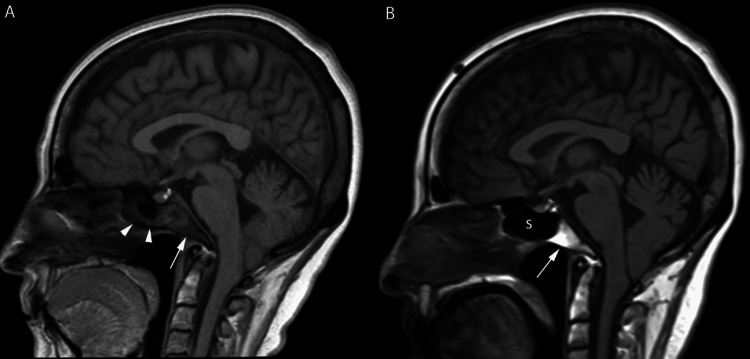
Magnetic resonance imaging of the head Sagittal T1 weighted images (A) demonstrate mild mucoperiosteal thickening in the sphenoid sinus (arrowheads in A) with abnormal signal intensity within the adjacent clivus (arrow in A). The abnormal signal intensity in the clivus becomes more apparent (arrow in B) with no significant sinus disease in the adjacent sphenoid sinus (s in B).
